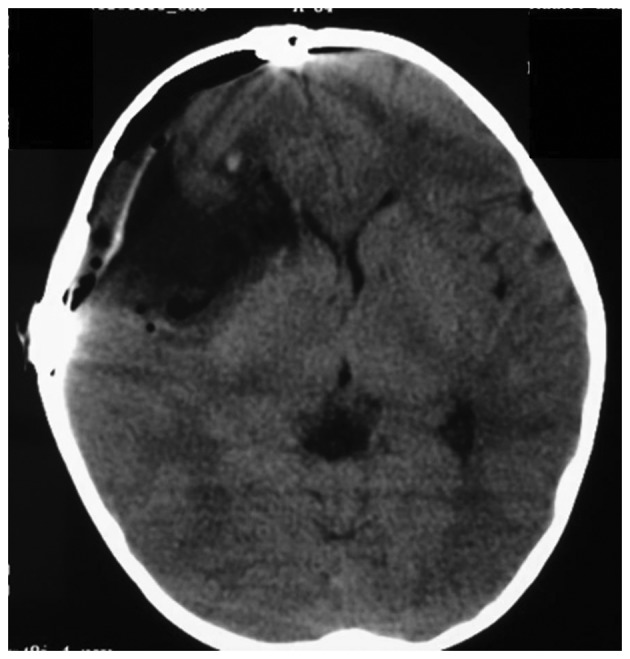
A CT scan was performed following the initial resection and showed gross total resection of the tumor. CT, computed tomography.
